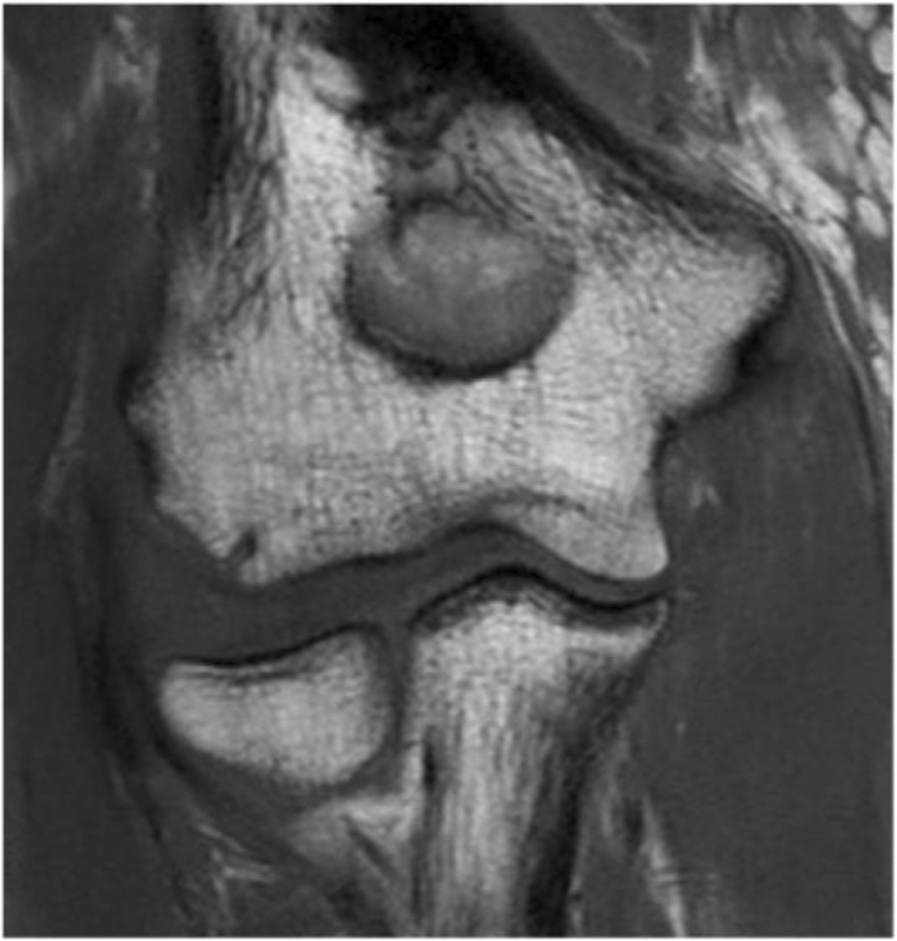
Complete tear of the LCL with radio-humeral joint incongruence in coronal view in MRI
